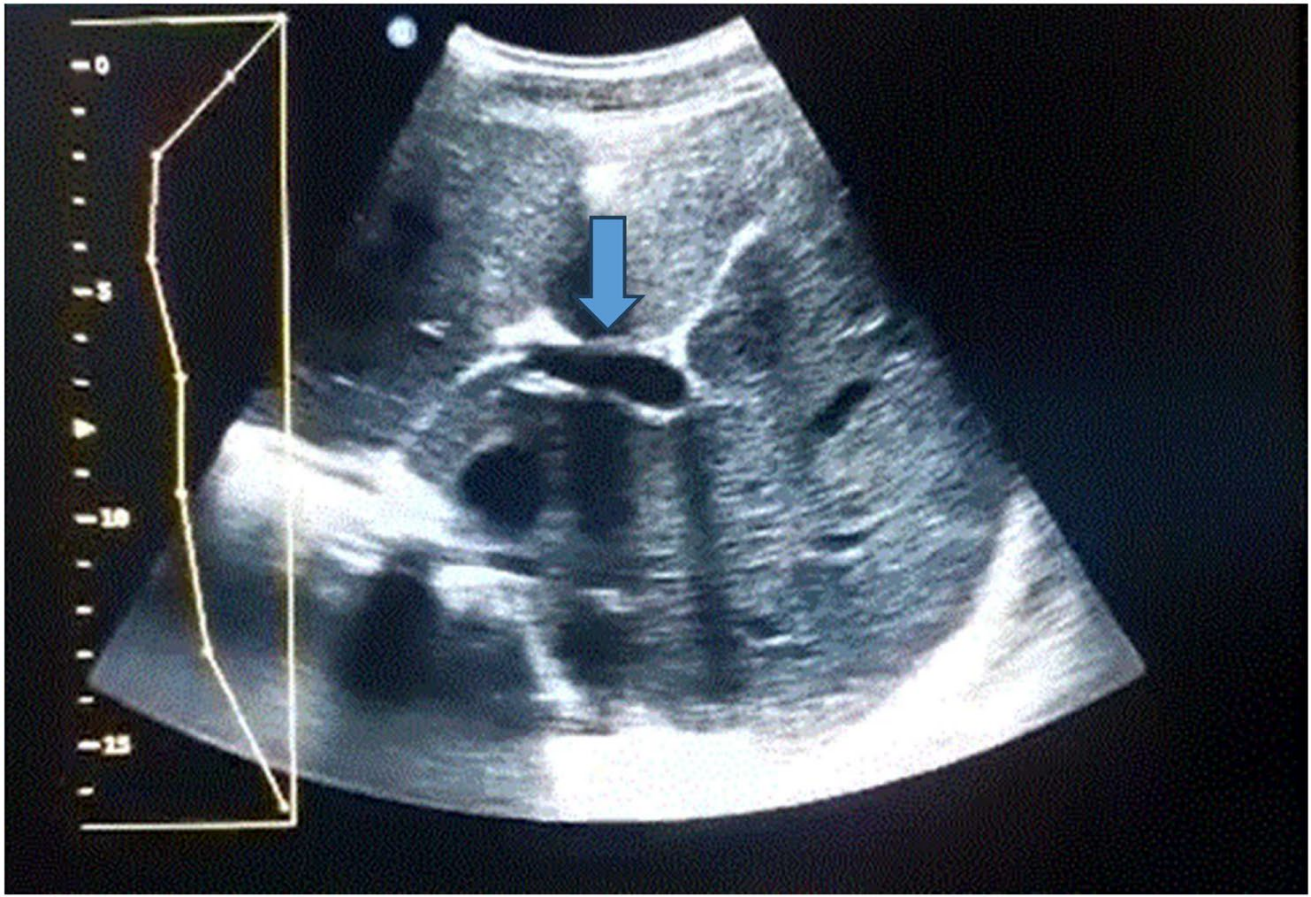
Abdominal ultrasound after 5 months of follow‐up.
